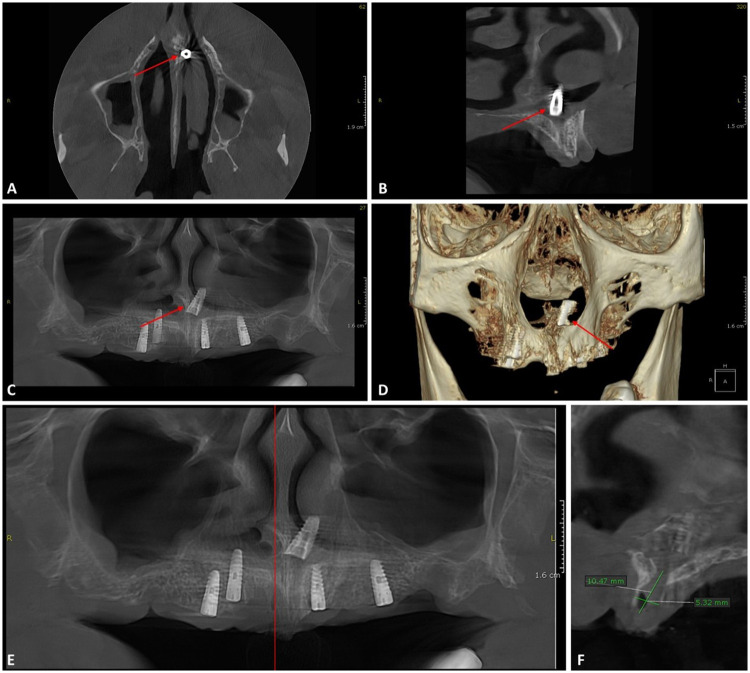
CBCT images showing the displaced dental implant in the left nasal cavity (red arrow): (A) axial view—implant crossing the midline and perforating the nasal floor. (B) Sagittal view—implant apex penetrating the nasal mucosa. (C) Coronal view—implant misalignment relative to adjacent implants. (D) 3D reconstruction—visualization of the implant within the nasal cavity. (E) Panoramic reconstruction indicating the section plane used for quantitative assessment (red vertical line). (F) Cross-sectional view at the level indicated in (E), demonstrating reduced residual ridge height (∼10.5 mm) and buccopalatal width (∼5.3 mm) at the implant site.
